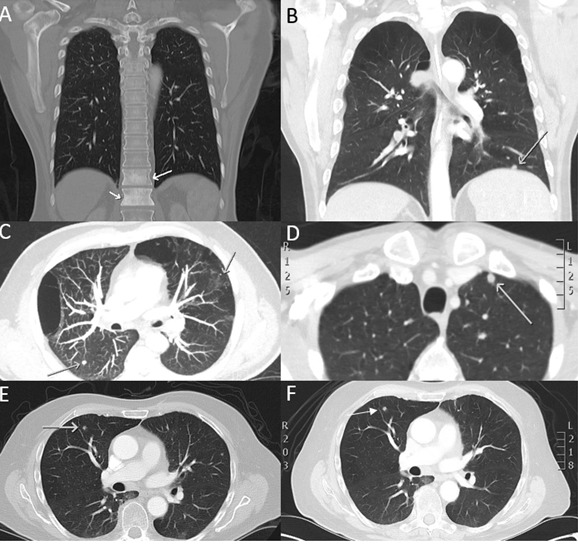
CT scan images of selected HNC patients presenting with suspicious HNC metastasis Bloods were taken upon presentation of these patients to clinic and CTCs assessed. Patient presenting with A. bony metastasis B., C., D. multiple suspicious lung metastasis E., F. lung nodule which increased in size over 37 days.
